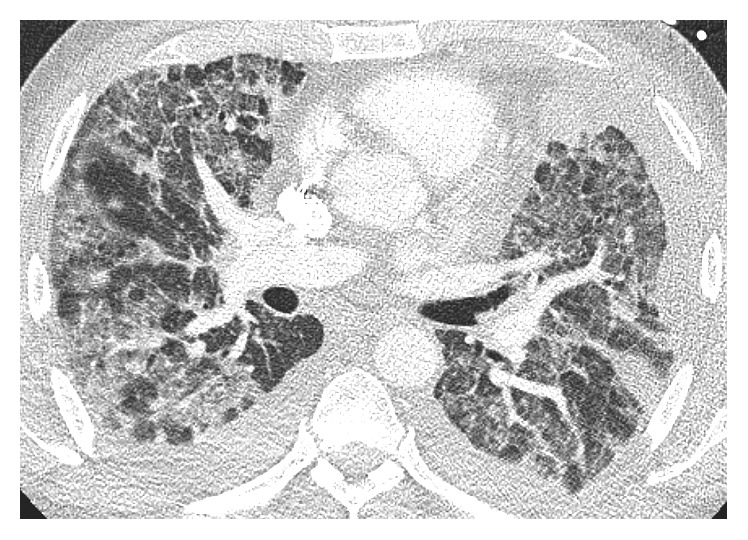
Chest CT showing diffuse ground glass opacities and new interlobular interstitial thickening.
